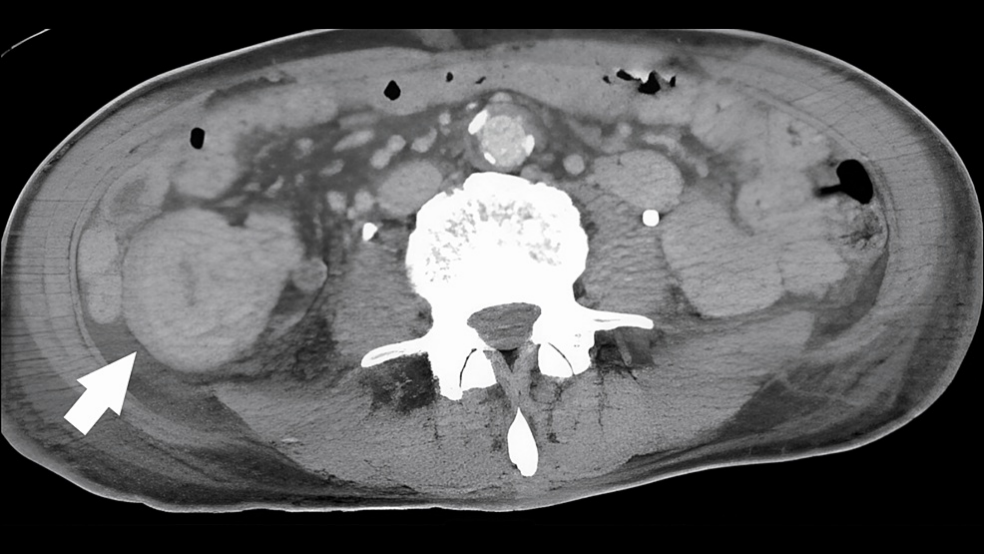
Abdominal computed tomography showing a mass in the right lower abdomen
